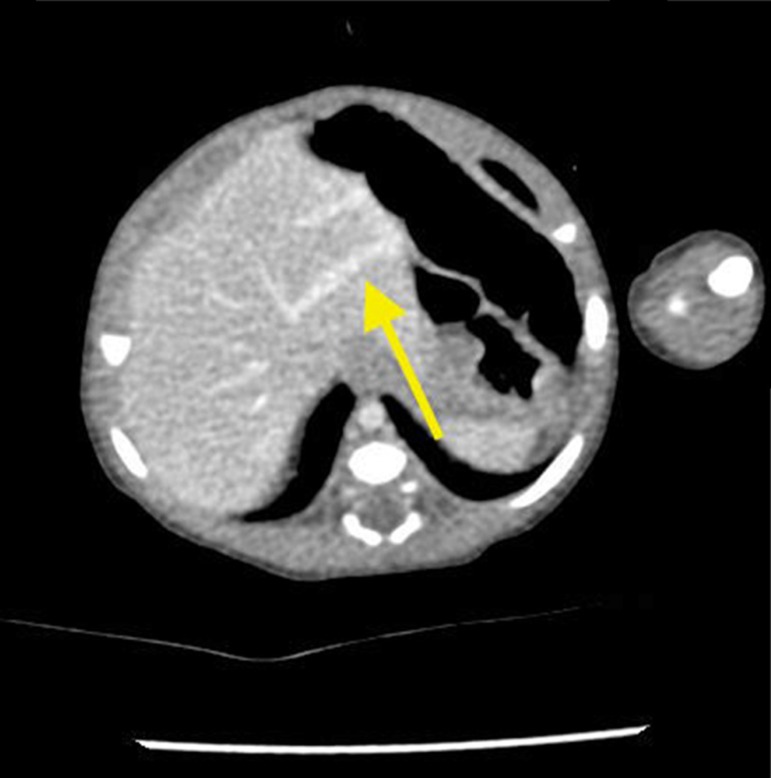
Abdominal CT angiography - venous phase. The arrow points to dilation of the intrahepatic portal vein, with portosystemic shunt.
